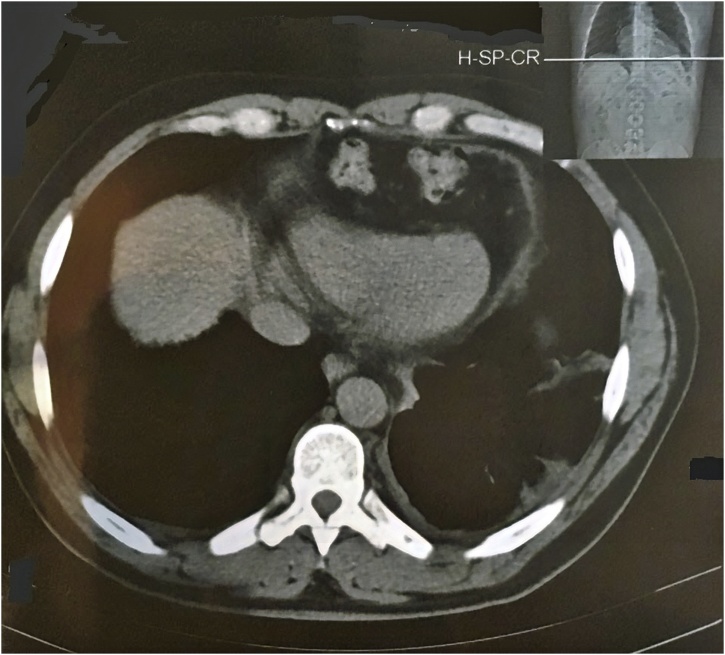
Computed Tomography (CT) scan revealed herniation of the transverse colon and omentum through a large anterior diaphragmatic defect into the pericardial sac.
